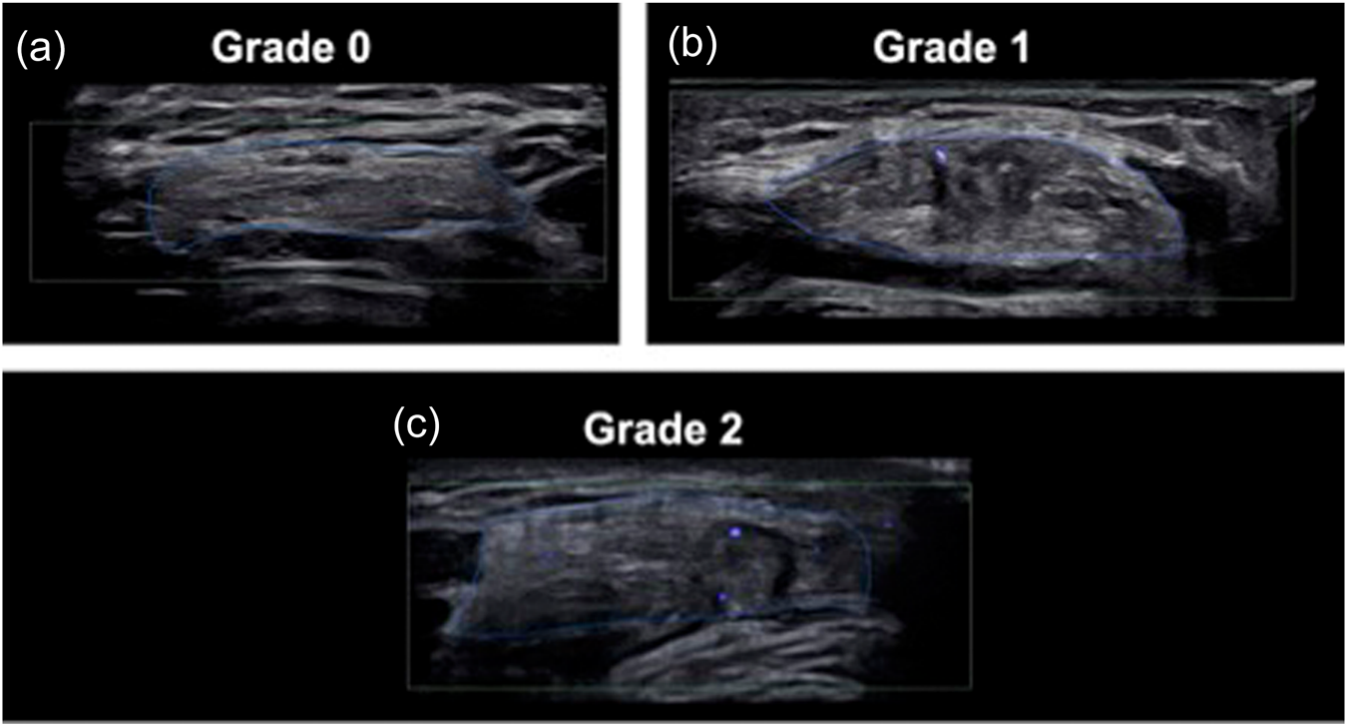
Evaluation of quadriceps tendon vascularity using superb microvascular imaging. The tendon outline is marked by a blue line. (a) Grade 0: No vascular signal. Ultrasound performed 2 years post‐operatively. (b) Grade 1: 1–2 spots of vascular signal (≤2 mm). Ultrasound performed 1 year post‐operatively. (c) Grade 2: More than 2 spots of vascular signal. Ultrasound performed 1 year post‐operatively.
